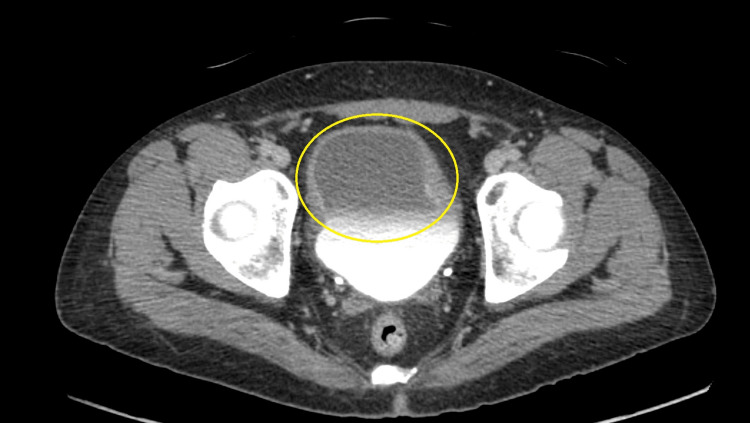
CT urogram of the patient The yellow circle marks the extensive and asymmetric bladder wall thickening noted
